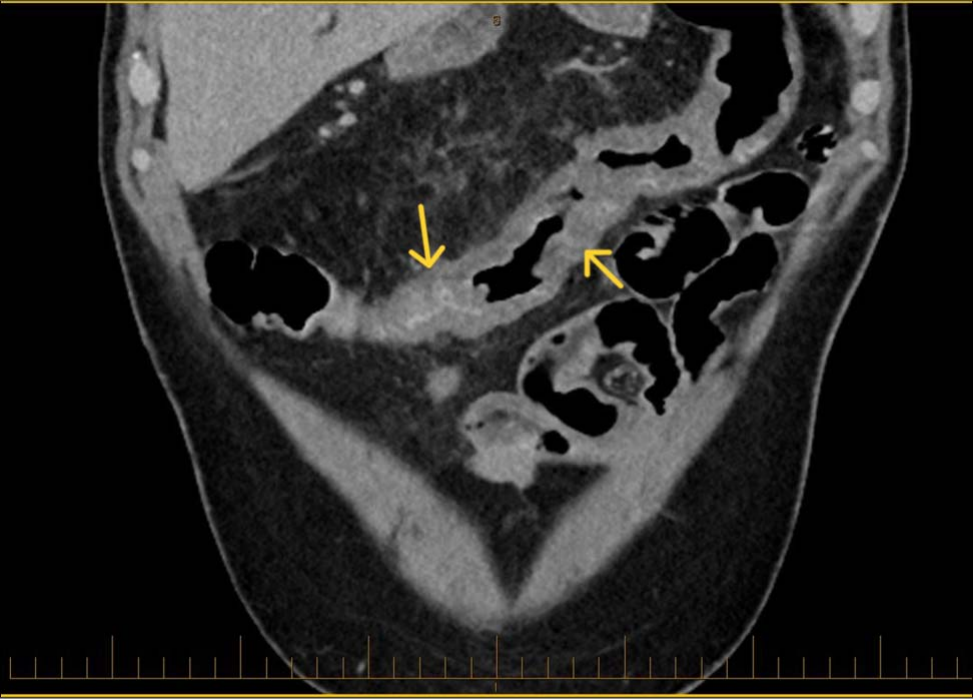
CT coronal view showing colitis. Arrows showing colon wall thickening/oedema likely reflecting varices.
